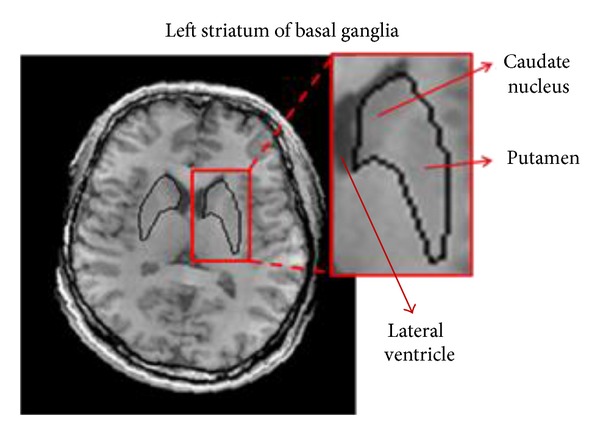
Anatomical structure of the striatum from the axial view of an MR T1-weighted image.
